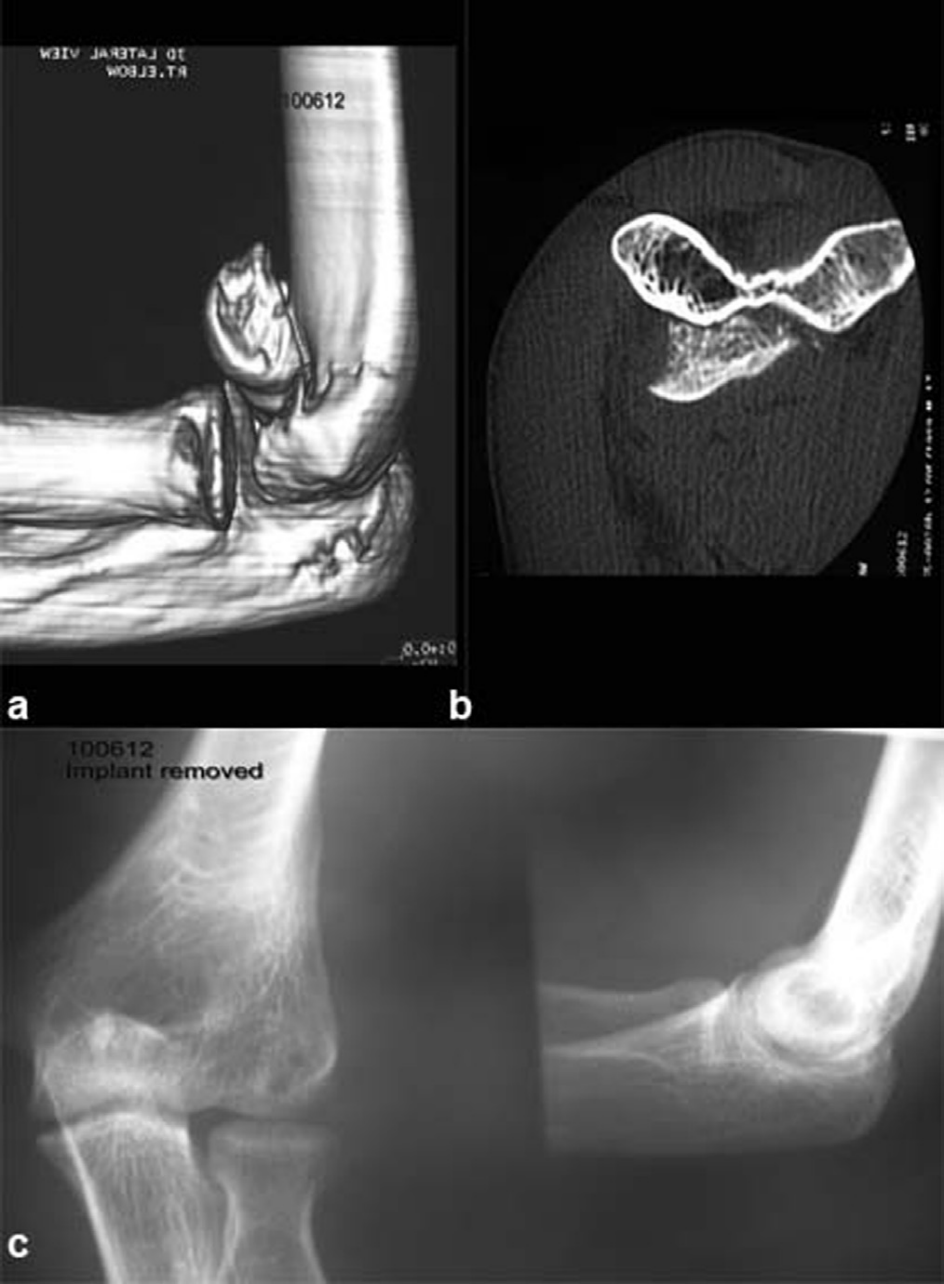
(a) 3D CT scan showing involvement of the trochlea. (b) CT scan axial section showing the involvement of the trochlea upto the trochlear groove. (c) X-rays after implant removal showing normal articular congruity
